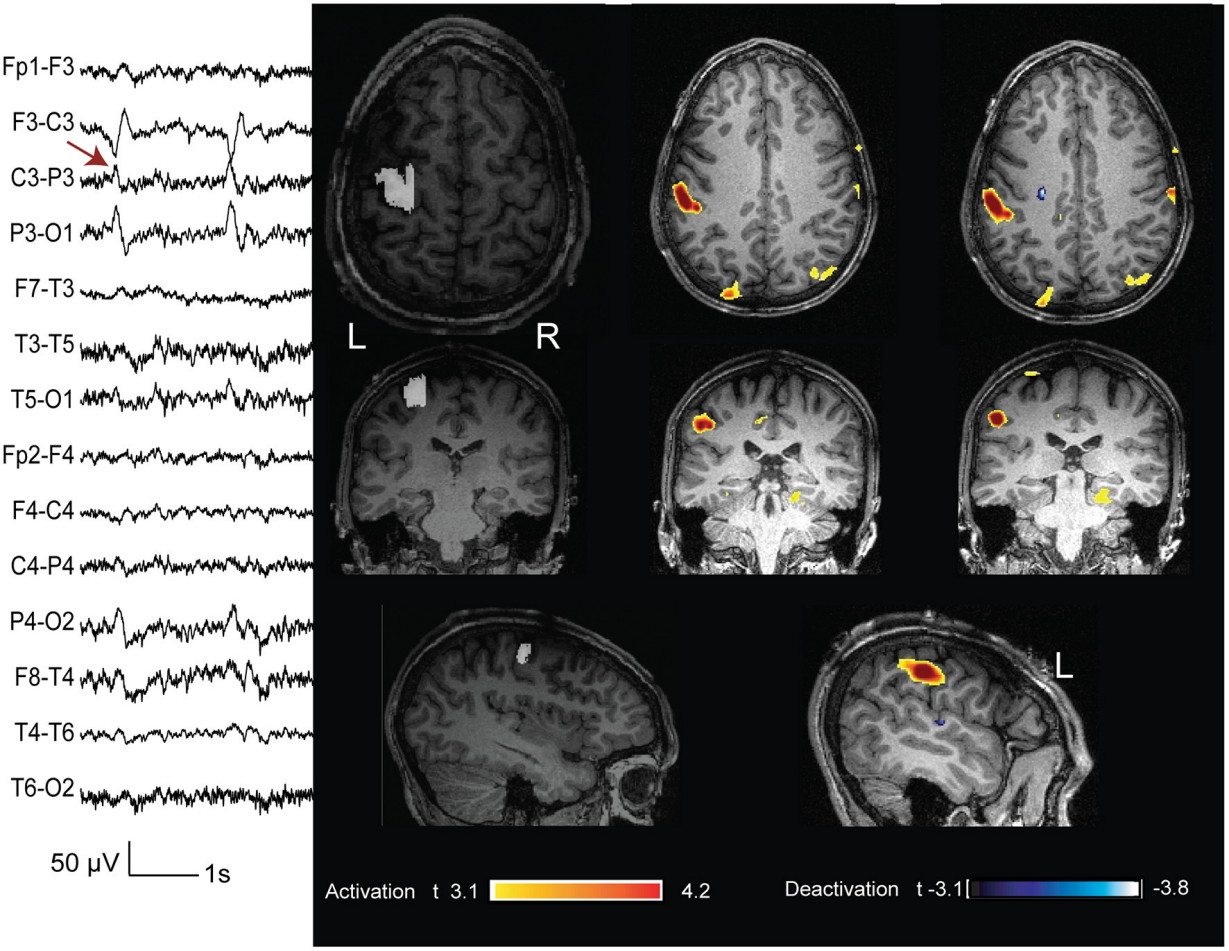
Twenty year old patient (case 8) with seizures characterized by a tingling sensation starting in the right hand digits and then propagating to the forearm, followed by right arm stiffness and jerks and sometimes secondarily generalization. The structural MRI did not show any lesion. The marked events were spikes with phase reversal at C3 (bipolar montage). The BOLD showed different clusters of activation: that with the highest *t*-value is in the postrolandic mid-sensory cortex. The intracerebral chronic electrocorticogram confirmed this finding. During surgery the pre-central gyrus was removed (white marking) and the histopatology revealed the presence of dysplastic cells. This case was considered as not concordant.
